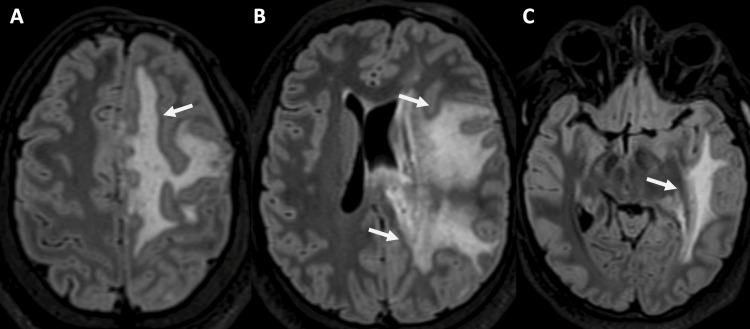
Volumetric T2 FLAIR axial images in the dorsoventral direction (A-C) show subcortical white matter signal abnormalities involving the left fronto-parieto-temporal lobe and the splenium of the corpus callosum (arrows)
